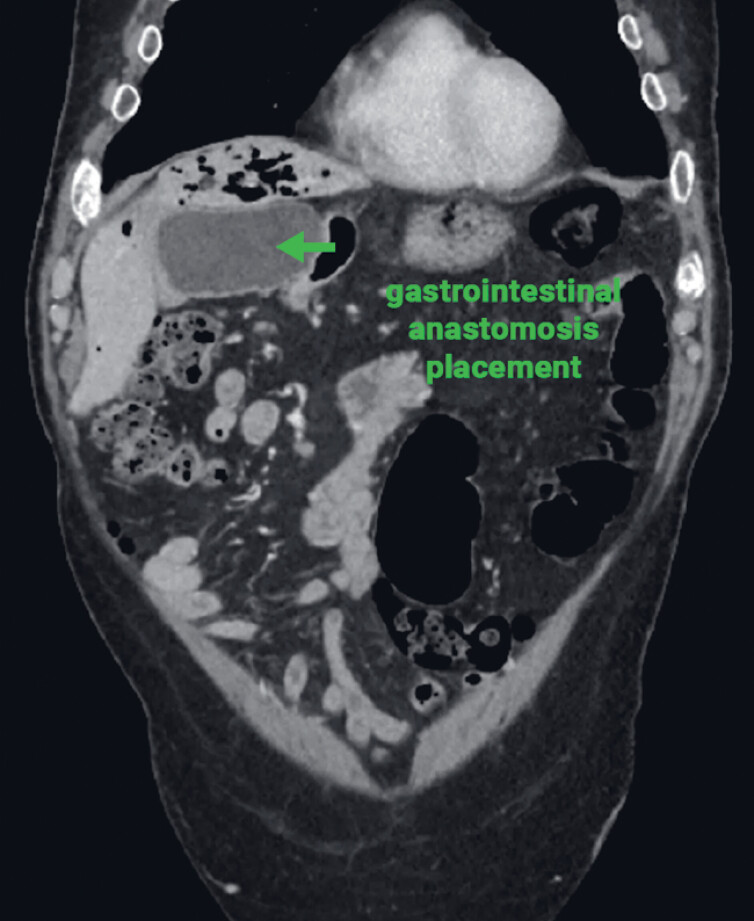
A preoperative CT scan showing the feasibility of the endoscopic ultrasound-guided placement of a LAMS between the gastric antrum and the afferent jejunal loop. CT, computed tomography; LAMS, lumen-apposing metal stent.
